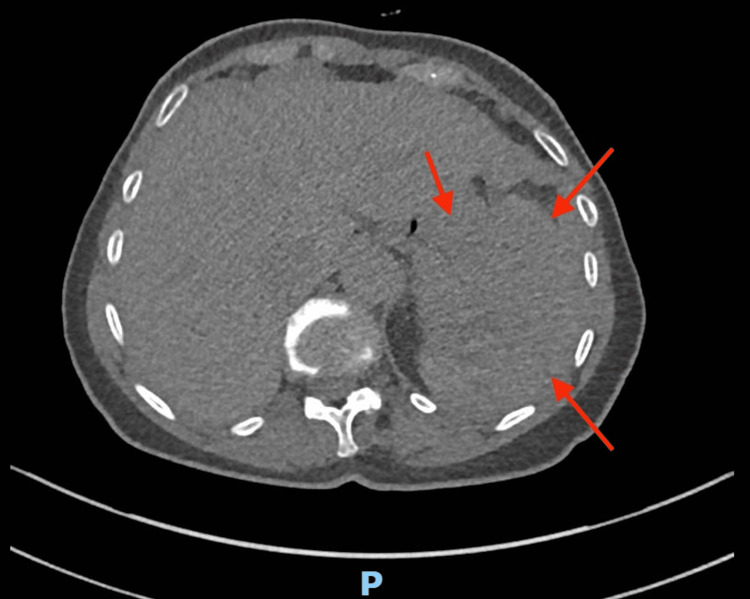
CT pulmonary angiography showing the upper pole of the spleen. Red arrows indicate areas reviewed for subtle contour irregularity on retrospective assessment. No definite abnormality was identified due to limited splenic coverage and non-splenic contrast timing.
